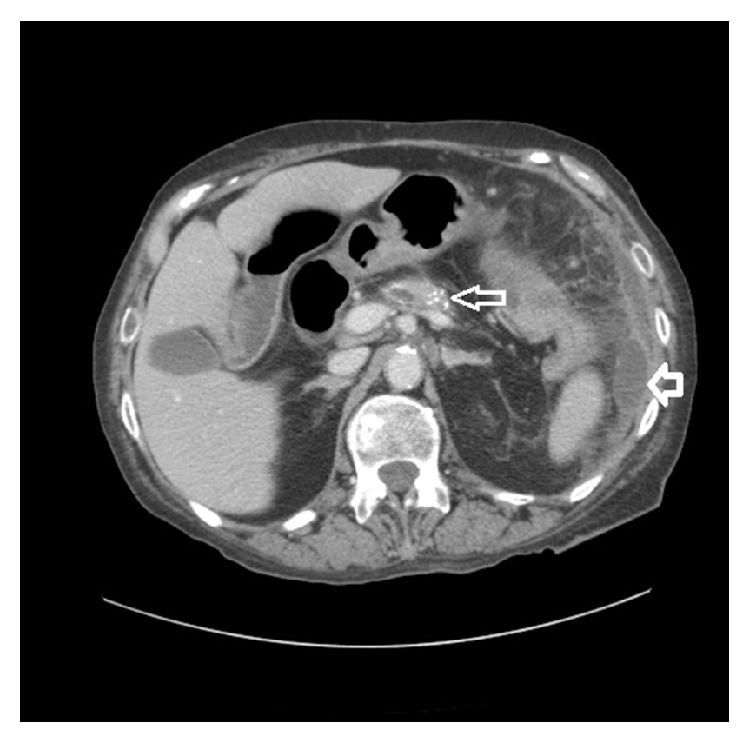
CT abdomen/pelvis done at outside hospital at time of admission: Fat arrow indicates fluid collection near the pancreatic tail and splenic flexure 3.5 cm. Skinny arrow calcification of the pancreas suggesting chronic pancreatitis.
